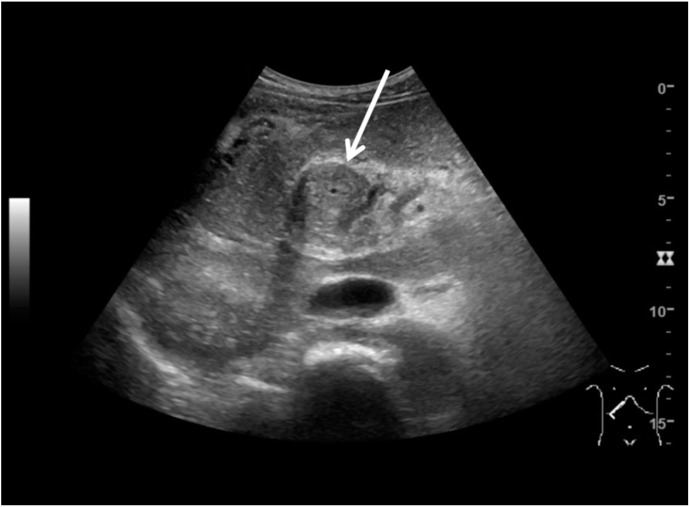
Ultrasound image of the abdominal mass (5.9 × 8.7 cm; arrow) in the right upper quadrant, surrounding the common bile duct.
